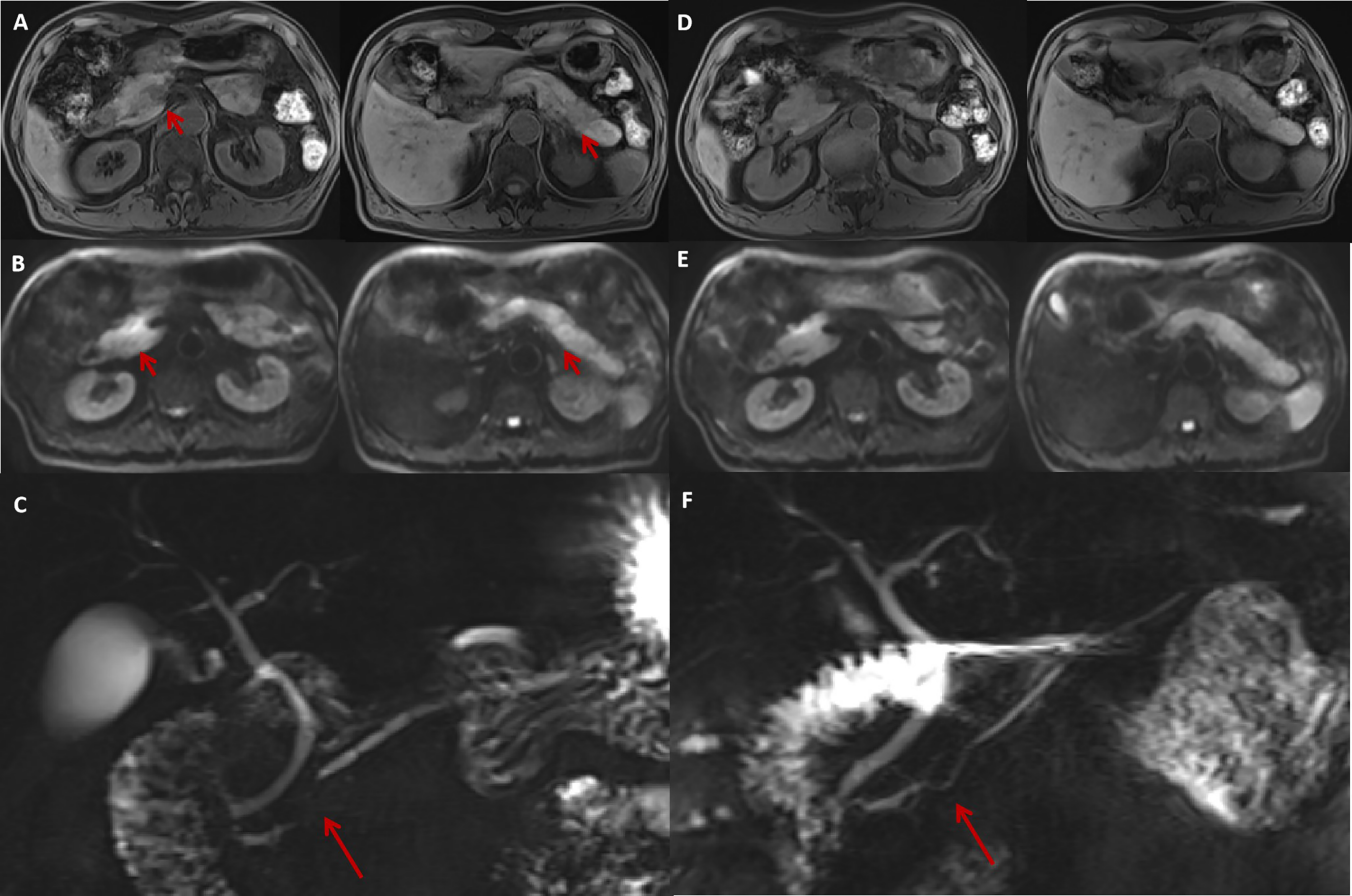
The MRI features of irAP and its improvement after treatment. The MRI at diagnosis revealed a slightly swollen pancreas with multiple nodular (A, arrow) and patchy abnormal signals with decreased intensity on T1WI sequences (A) and increased intensity on DWI (B, arrow) located in the pancreatic head and tail. MRCP showed segmental stenosis of the MPD in the pancreatic head (C, arrow). After 1 month of prednisolone treatment, the swelling reduced and the abnormal signals decreased on T1W1 (D) and DWI (E) sequences. The MPD stenosis also resolved (F, arrow). irAP, immune-related acute pancreatitis; MRI, magnetic resonance imaging; T1W1, T1-weighted image; DWI, diffusion-weighted imaging; MPD, main pancreatic duct; MRCP, magnetic resonance cholangiopancreatography.
